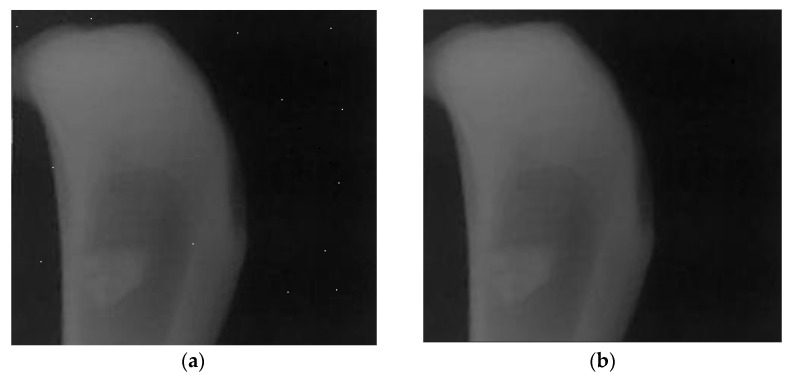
Salt and pepper noise reduction using a median filtering technique; (a) original s-X-ray detected image, (b) image result after taking the median filtering technique.
